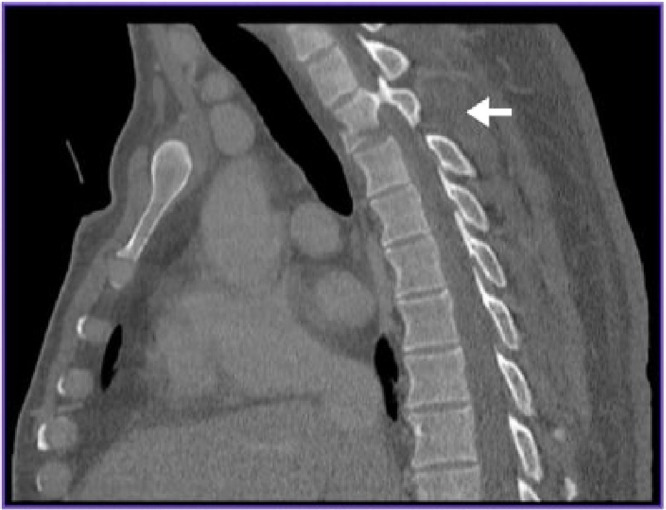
Sagittal computed tomography (CT) image with T2 vertebral body fracture with locking of the right T2-T3 facet joint (Arrow).
